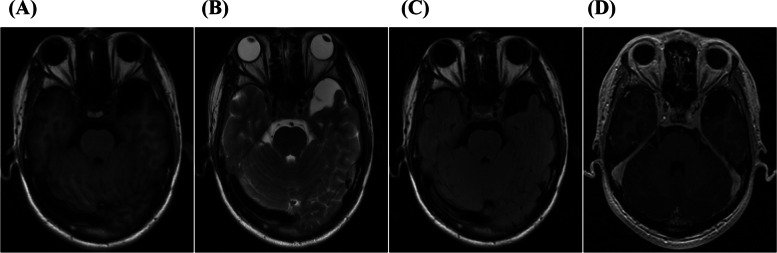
(A), (B), (C), (D) represent T1WI, T2WI, FLAIR, and post contrast 3D-BRAVO images. The MRI imaging of his brain was normal except for a CSF-containing arachnoid cyst on the left temporal lobe
